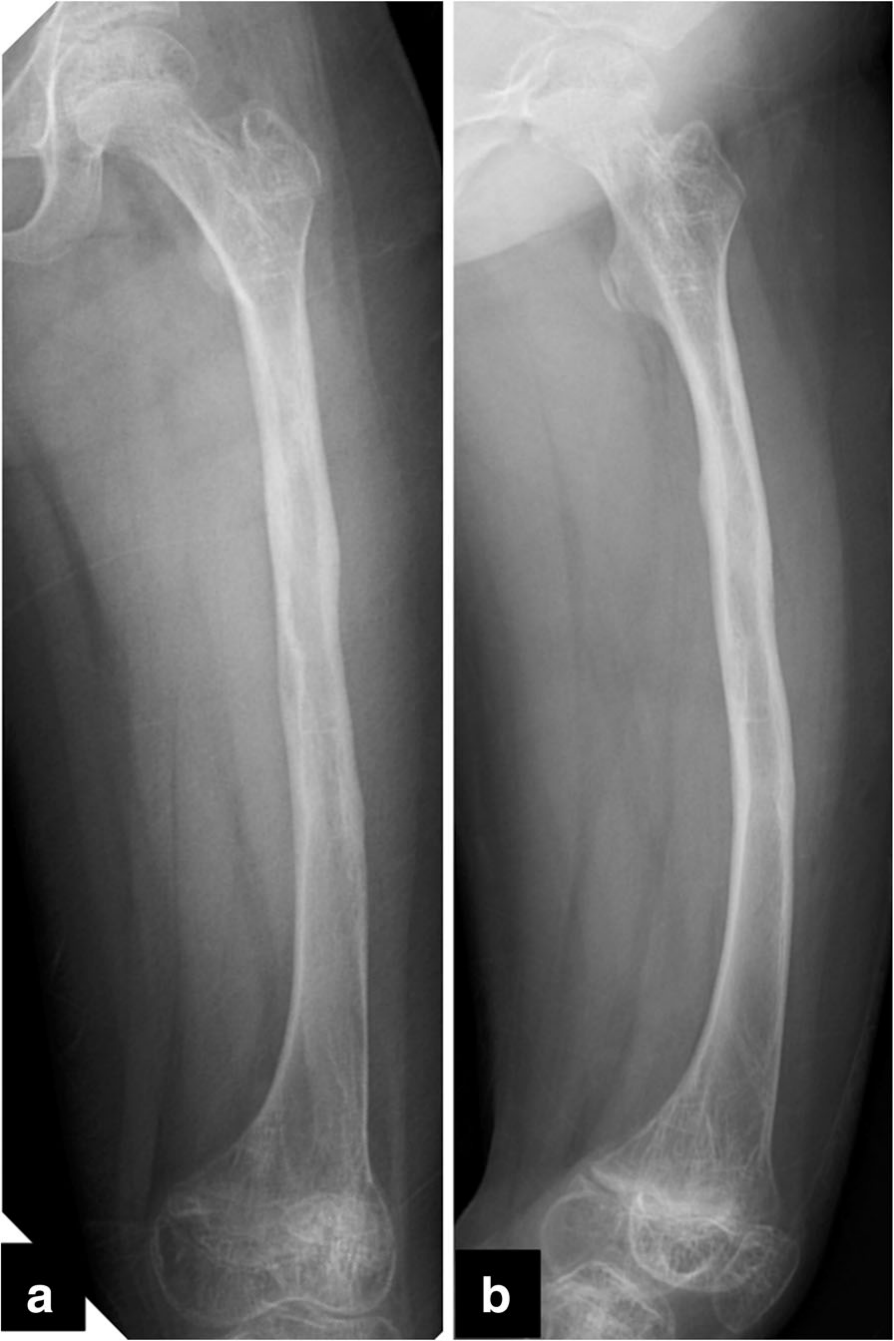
Radiographs of the left femur after the revision surgery after the removal of Ender nail (5-month after the nail insertion) showing solid bony fusion of all fractures. a the anteroposterior view, b the lateral view
